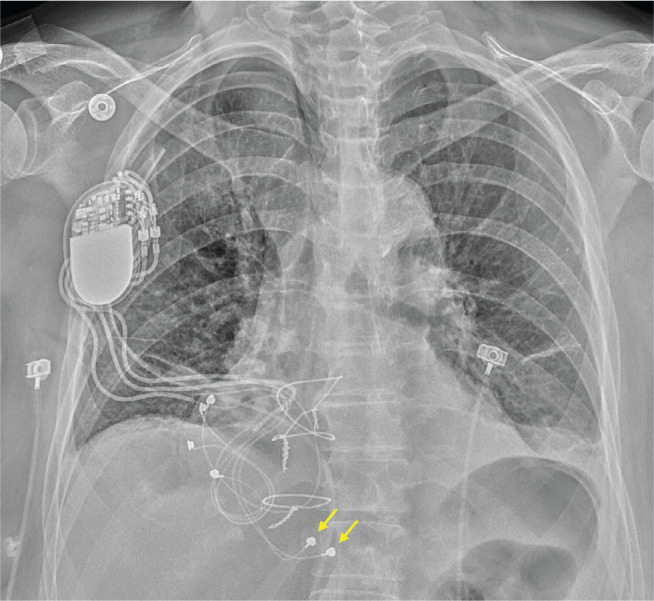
Frontal chest radiograph of a patient with a completely epicardial dual-chamber pacemaker system with leads overlying the right atrium and right ventricle (arrows).
